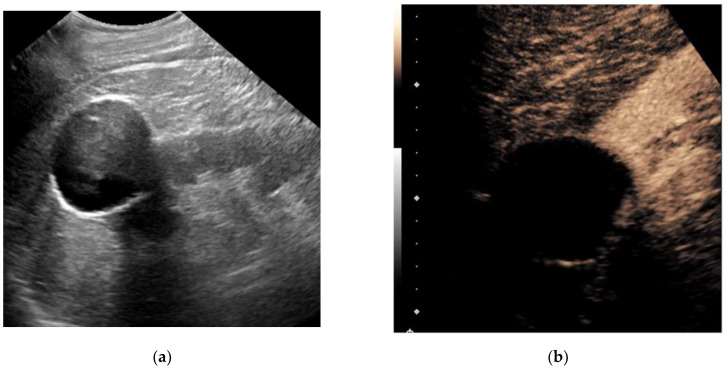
A 79-year-old man with a history of bladder cancer undergoing evaluation for hydronephrosis. Grayscale ultrasound image of the left kidney in the longitudinal orientation (a) shows an exophytic hypoechoic mass containing internal low-level echos. Following an intravenous injection of 1.8 cc Lumason ultrasound contrast, a contrast-enhanced ultrasound image focused at the upper pole (b) revealed the mass was completely non-enhancing (devoid of signal), which is diagnostic for a simple cyst. No further follow-up was necessary.
